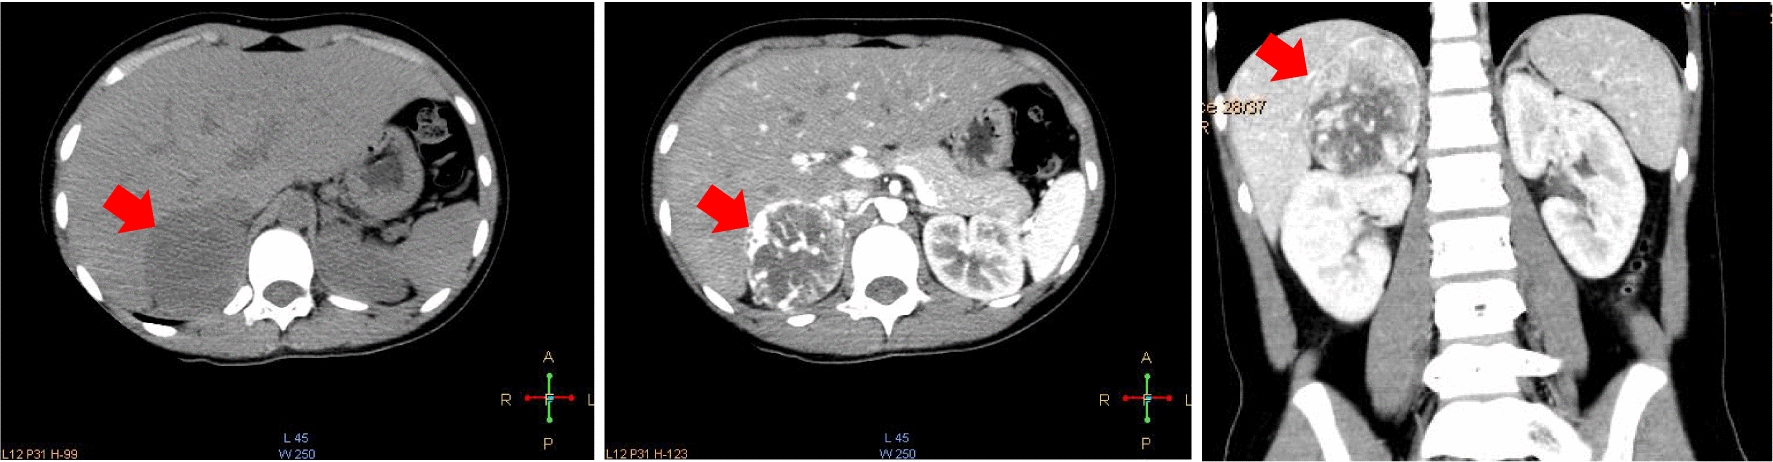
Transverse section and coronal section of CT scans indicated right pheochromocytoma, which were labelled as red arrow. CT scans indicated that tumor enhancement and central necrosis were obvious
